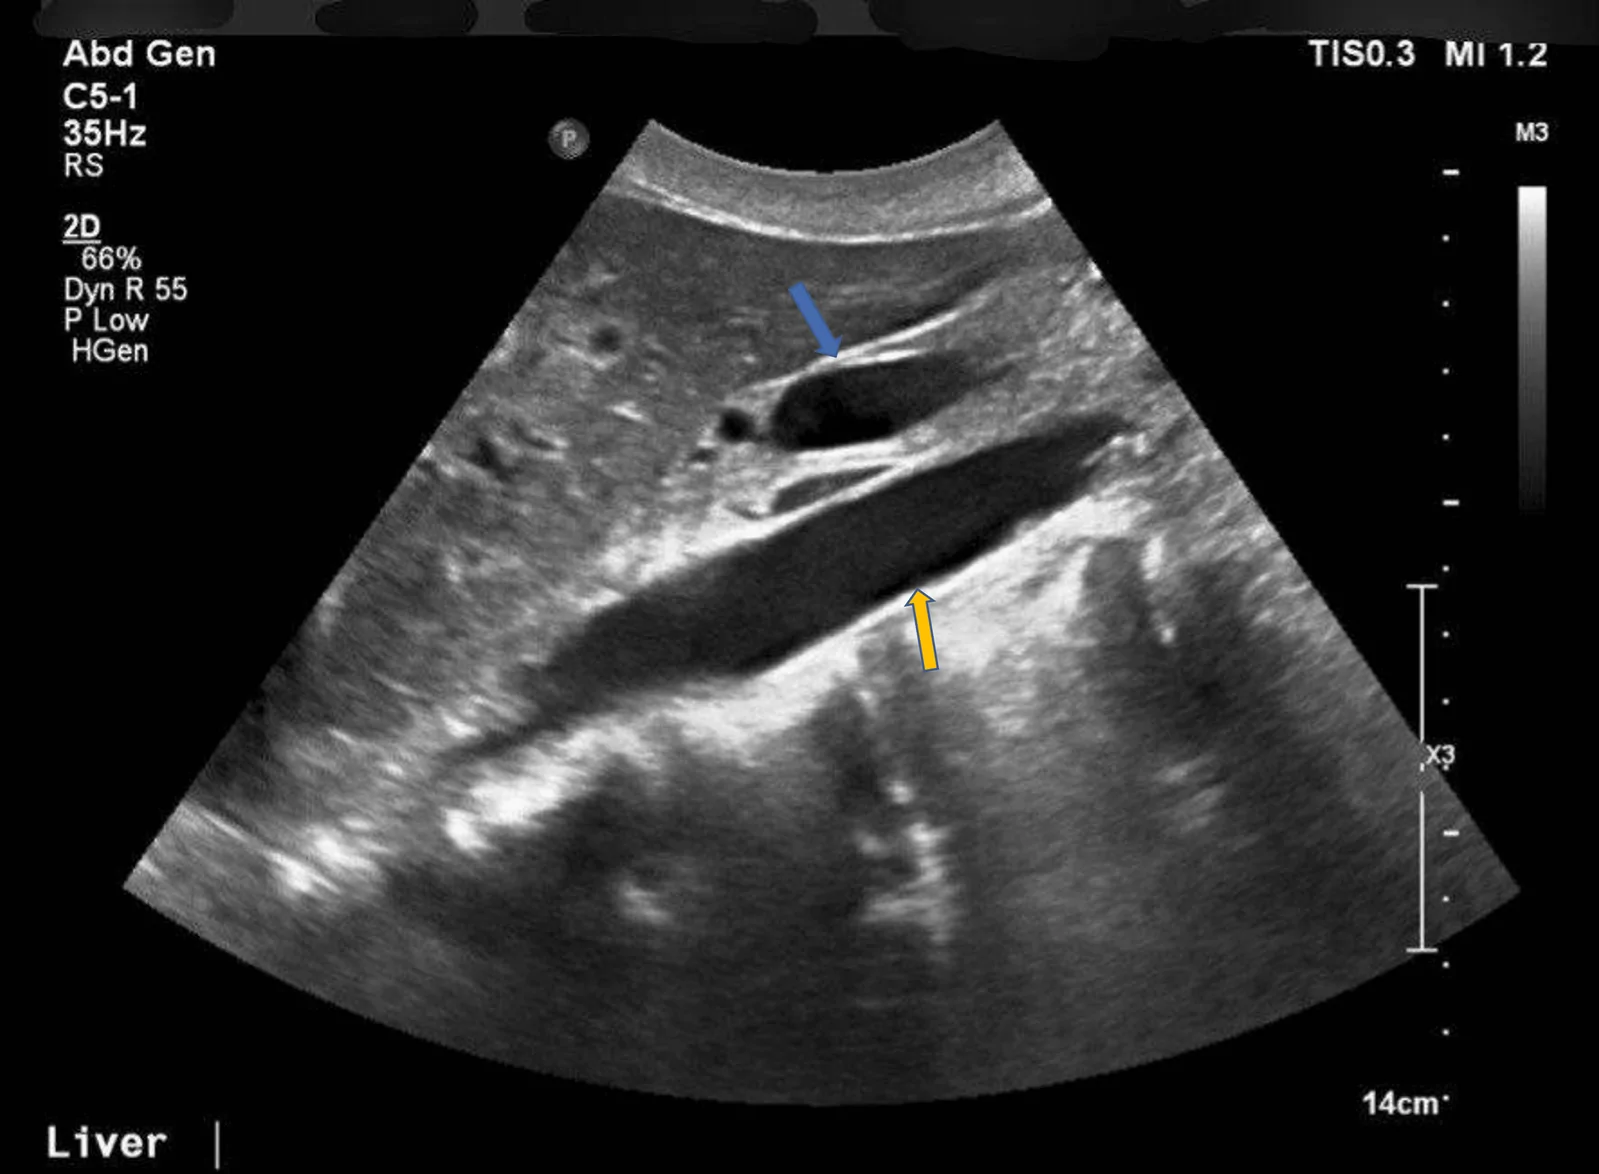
Abdominal ultrasound. Blue arrow: Engorged intrahepatic vein. Orange arrow: Dilated hepatic vein/inferior vena cava. Findings are consistent with systemic venous congestion in the context of dengue infection.
